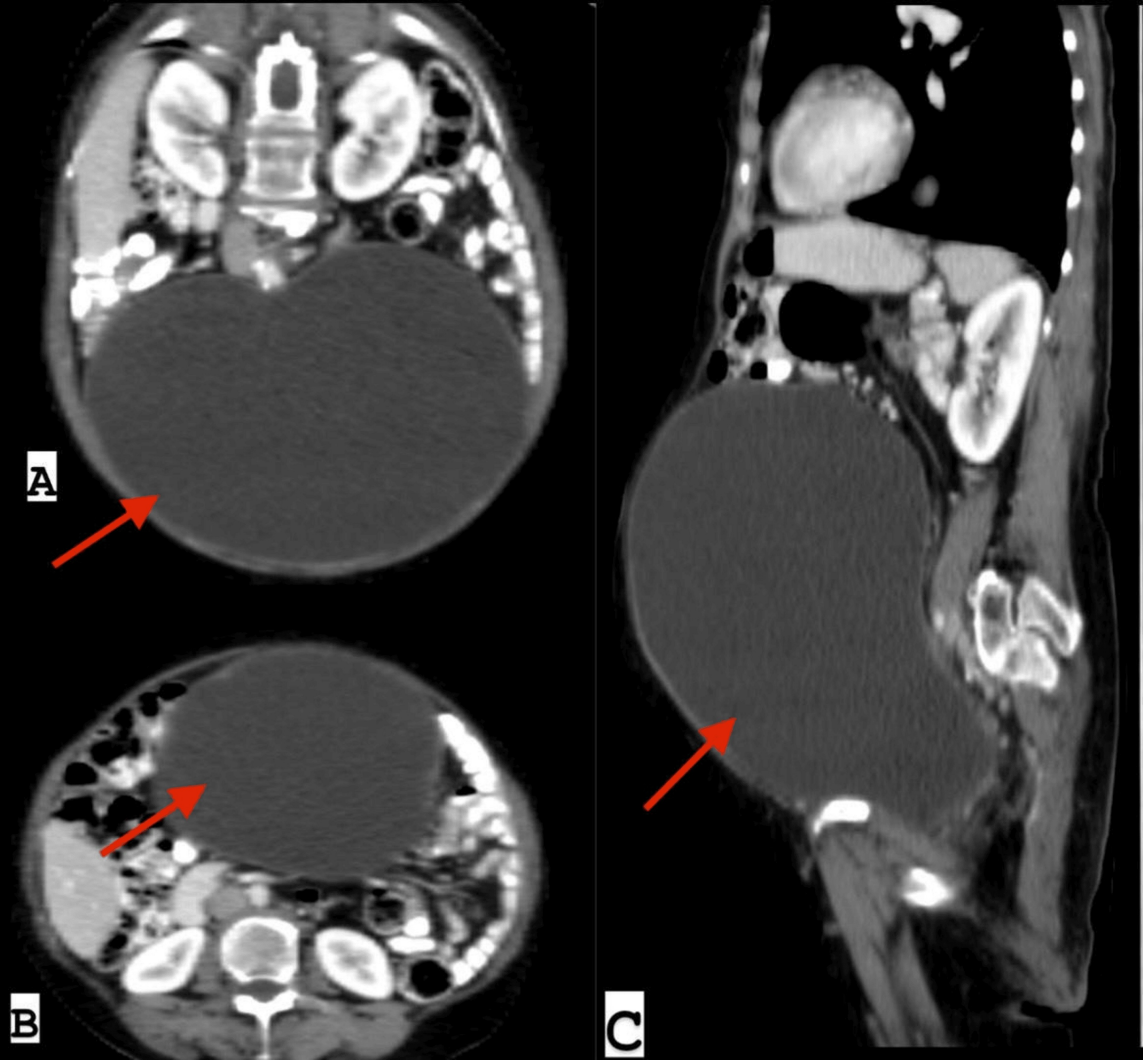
CT scan findings. A: Coronal oblique CT scan with IV and oral contrast showing hugely distended urinary bladder, reaching the level of kidneys. The bladder wall is smooth without irregularities. B: Axial CT scan with IV and oral contrast showing hugely distended urinary bladder, compressing other abdominal organs. C: Sagittal CT scan with IV and oral contrast showing the bladder replacing and compressing the small bowel, reaching up to the stomach level.
